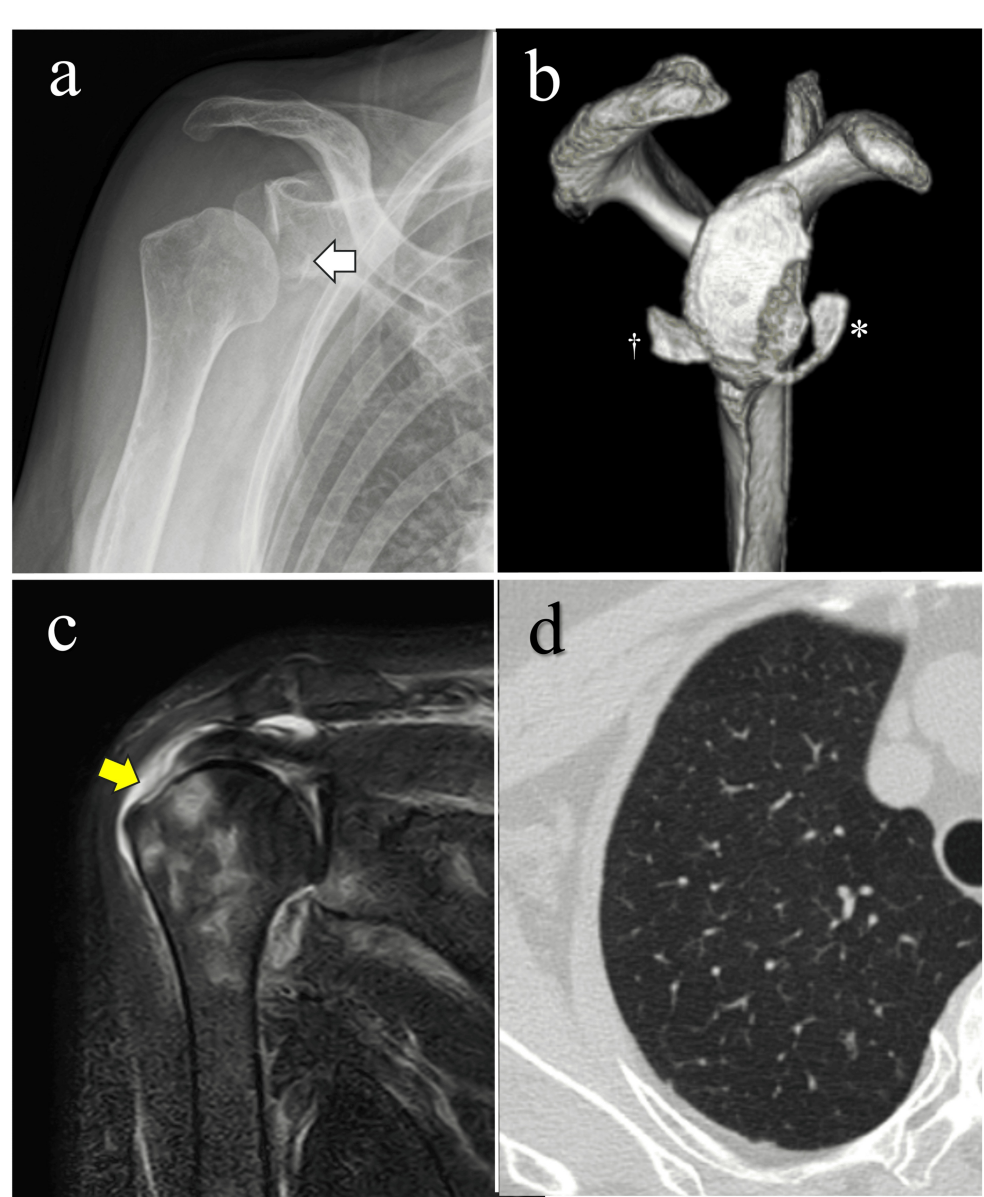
Preoperative imaging findings (a) Plain radiograph showing a glenoid bone defect (arrow) with poor joint congruency. (b) Three-dimensional computed tomography (CT) demonstrating a large bony Bankart lesion (asterisk) and a glenoid bone fragment (dagger). (c) Magnetic resonance imaging (MRI) revealing a full-thickness tear of the supraspinatus tendon (yellow arrow). (d) Chest CT showing no evidence of emphysematous changes.
